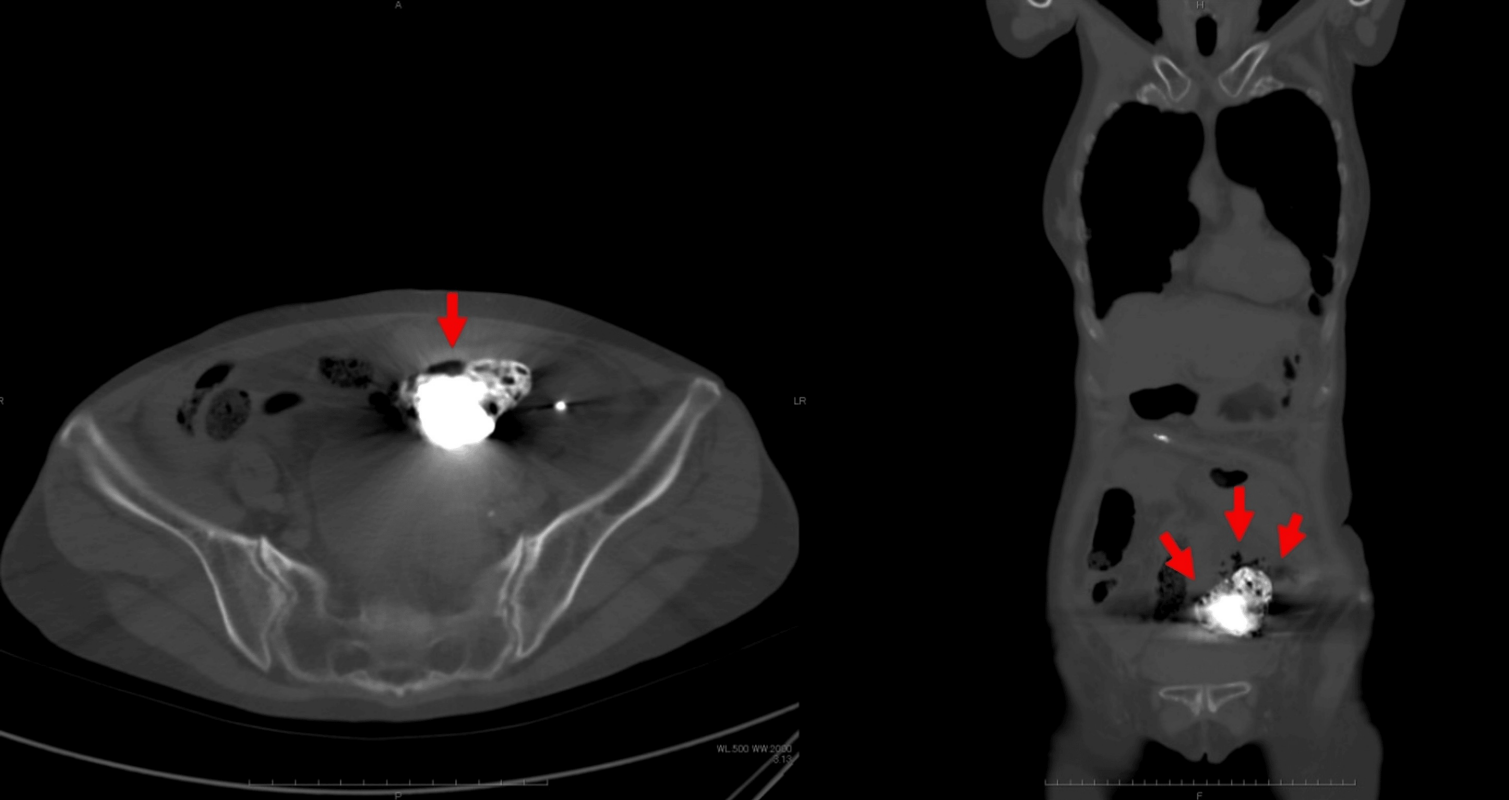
Plain abdominal CT scan A plain abdominal CT scan (axial view, left; coronal view, right) demonstrates sigmoid colon perforation with extravasation of barium contrast medium, free intraperitoneal air (red arrow), and a localized intra-abdominal abscess.
